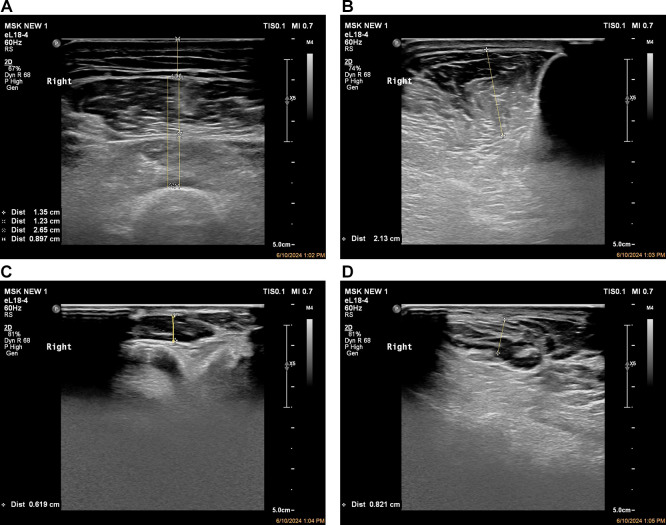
Quantitative musculoskeletal ultrasound assessment of lower limb muscles showing standardized probe position and muscle thickness measurement. (A) Measurement of the thickness of the anterior quadriceps muscle.
